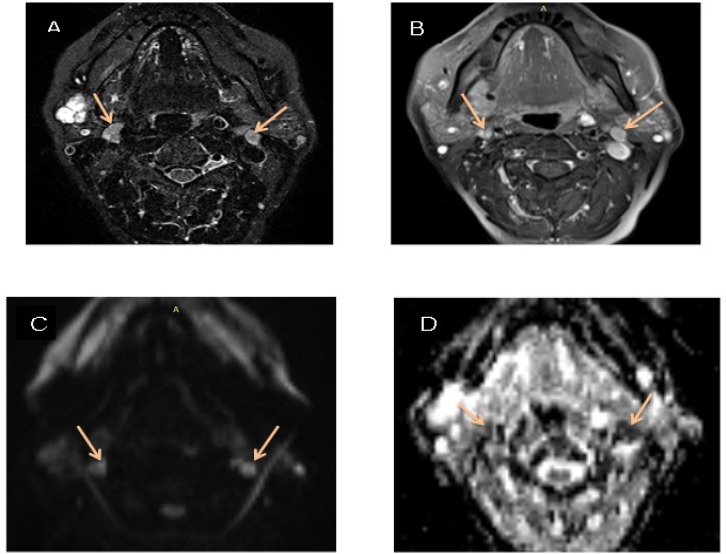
A 63 Year-Old Female with Parotid Mass. A, STIR shows two enlarged right and left neck LNs; B, T1W post contrast shows enhanced LNs; C, DWI shows high signal intensity; D, ADC displays restricted diffusion of LNs on ADC (0.883 × 10^-3^ mm^2^/s)
